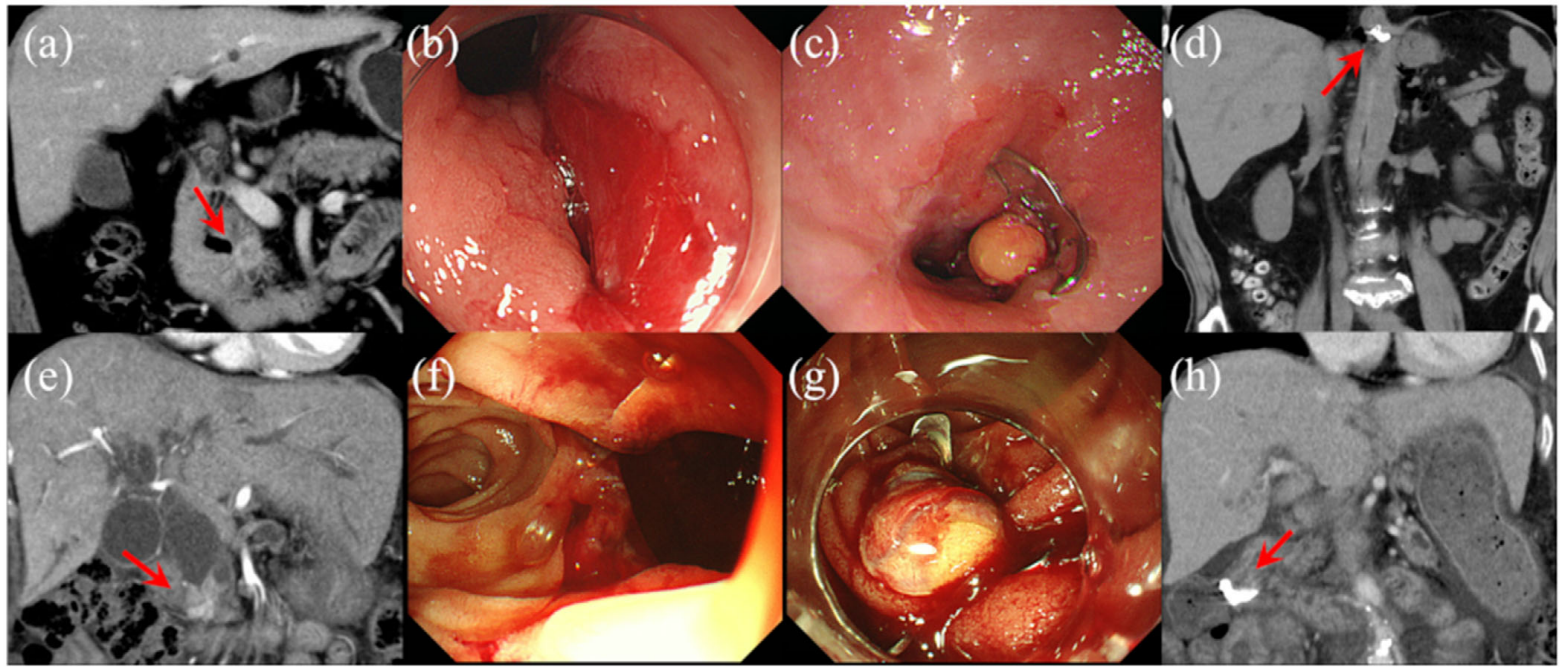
Case 3 (upper figure) and case 4 (lower figure). (a) Pancreatic head tumor on computed tomography (CT) (red arrow). (b) A large perforation, measuring approximately 12 mm in diameter, occurred in the posterior wall of the esophagogastric junction. (c) The wound filled with fatty tissue after placement of an over‐the‐scope clip (OTSC). (d) The OTSC detected on CT (red arrow) after the endoscopic repair. (e) Distal bile duct stenosis on CT (red arrow). (f) A large perforation, measuring approximately 20 mm in diameter, occurred in the posterior wall of the second part of the duodenum. (g) The wound filled with the omentum after placement of an OTSC. (h) The OTSC detected on CT (red arrow) after the endoscopic repair
